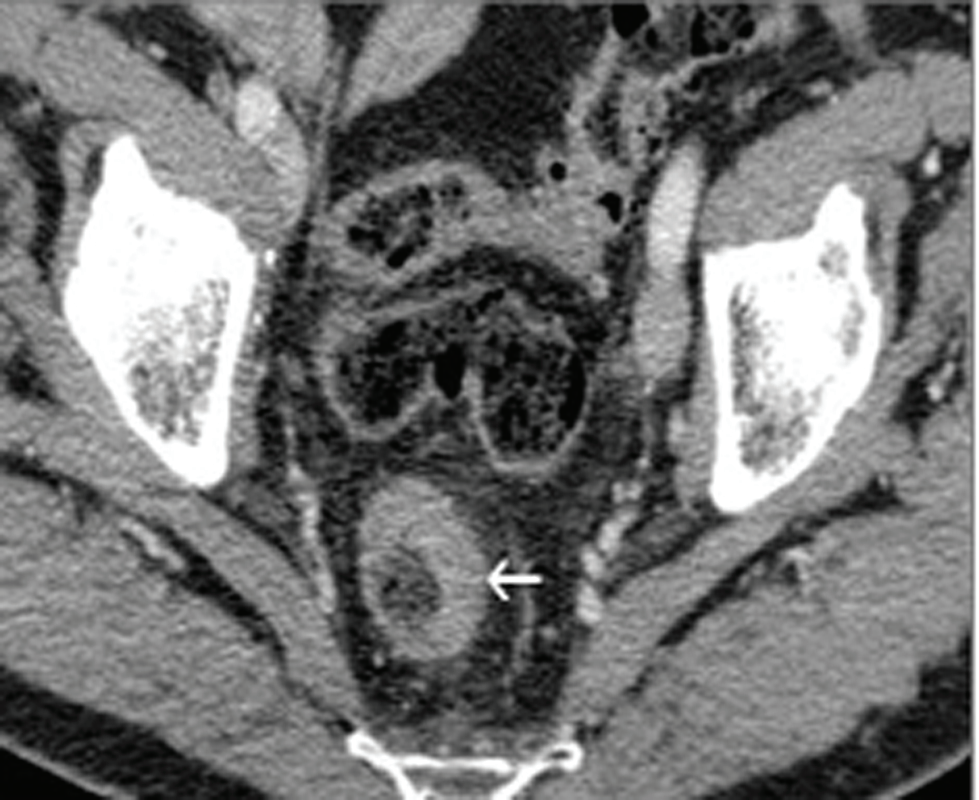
Transversal view of abdominal CT scan. Circumferential thickening of the rectal wall (arrow).
